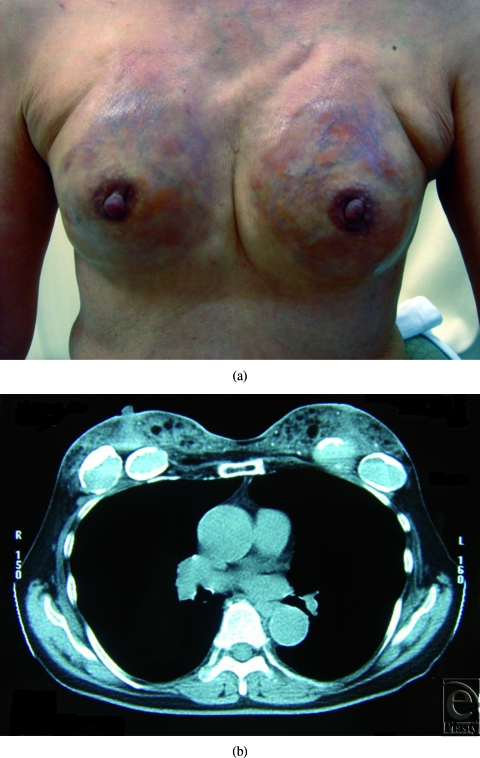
(*Left*) Preoperative appearance of the patient. (*Right*) Computed tomography revealed radiopaque images in the superficial layer and radiolucent images with eggshell-like calcification in the deep layer.
